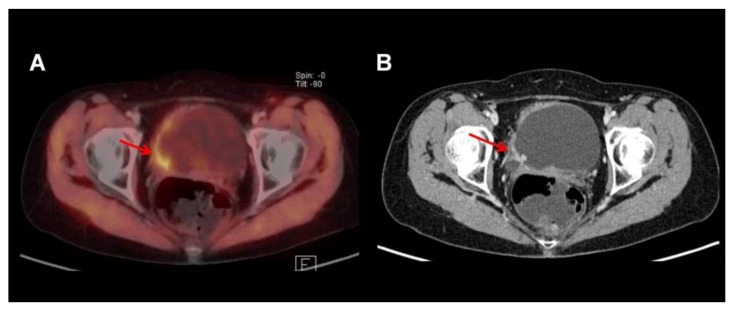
Bladder tumor with tracer uptake in the right posterior-lateral bladder wall as visualized by ^11^Choline-PET/CT. Note that there is excellent delineation of tumor and bladder lumen due to the fact that ^11^C-Choline is usually not excreted by the urine (A: fused dataset). However, anatomical detail is superior in the contrast enhanced CT part of the PET/CT (B). Note the hypervascularized tumor involving the right ureteral orifice with consecutive dilatation of the right ureter.
